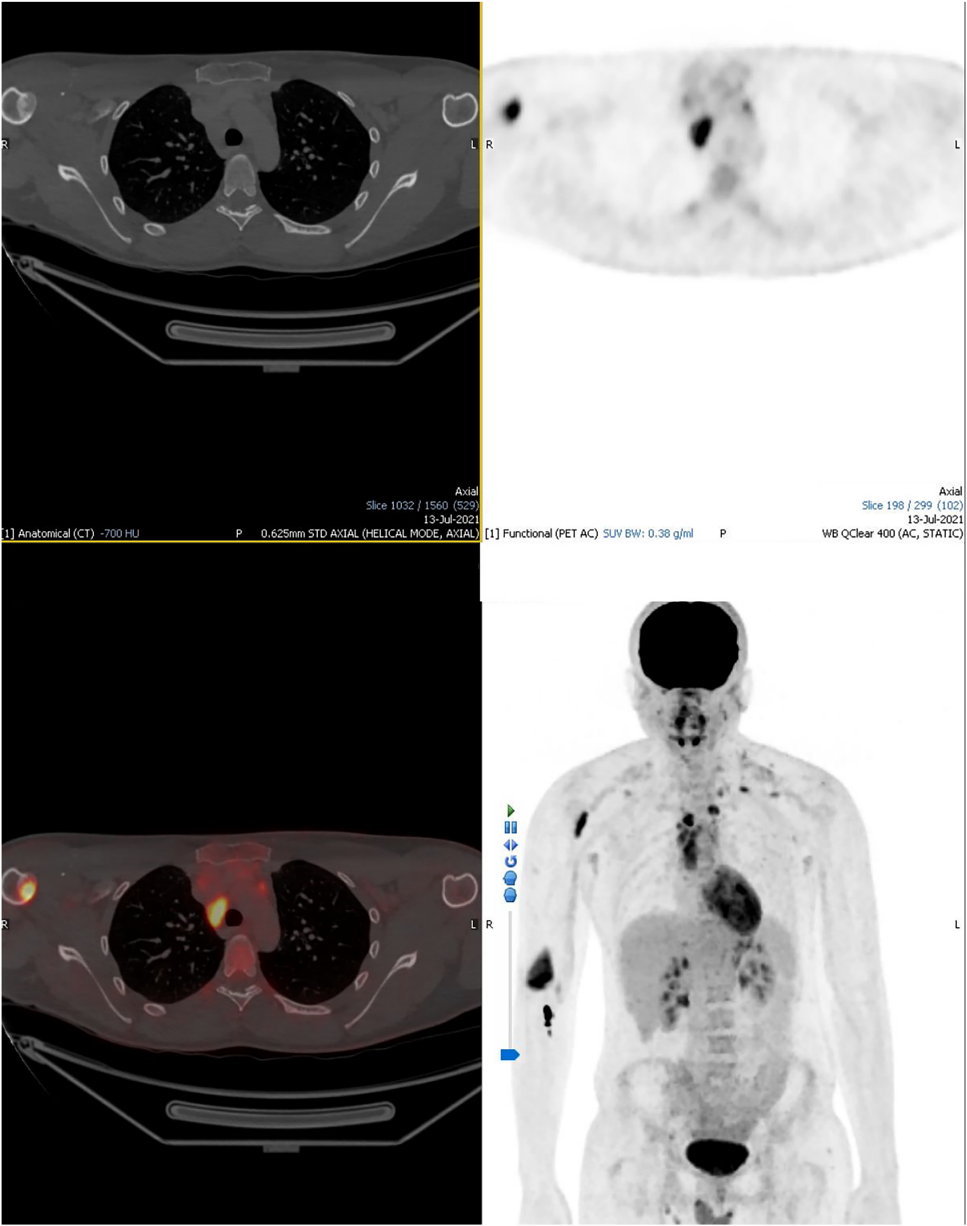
Initial staging PET ‐ CT demonstrating stage 4 disease.
